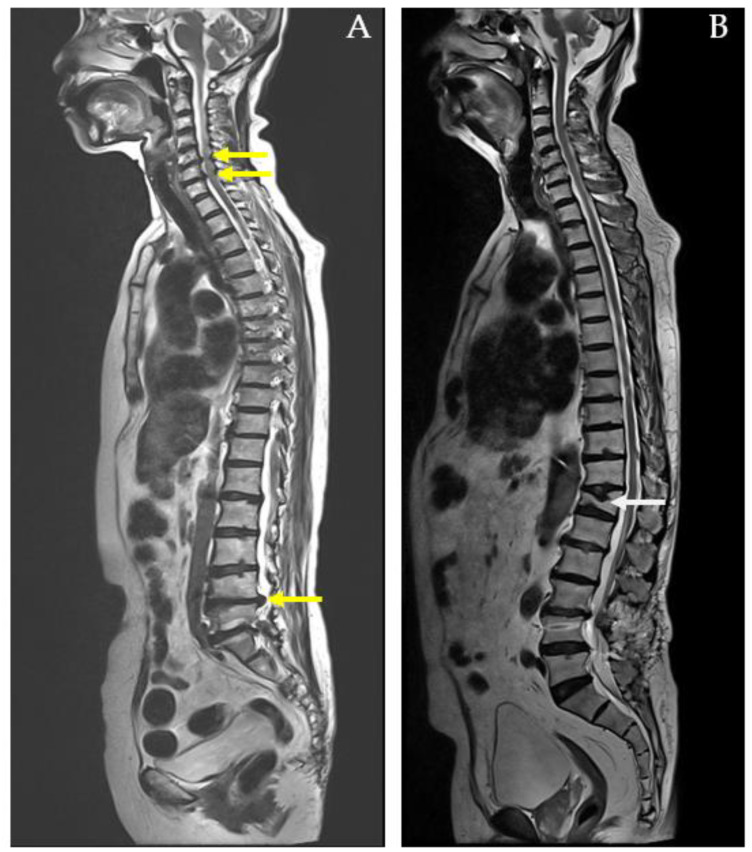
MRI whole-spine imaging demonstrating herniated intervertebral discs (HIVDs) indicated by yellow arrow in (A) and compression fracture indicated by white arrow in (B).
